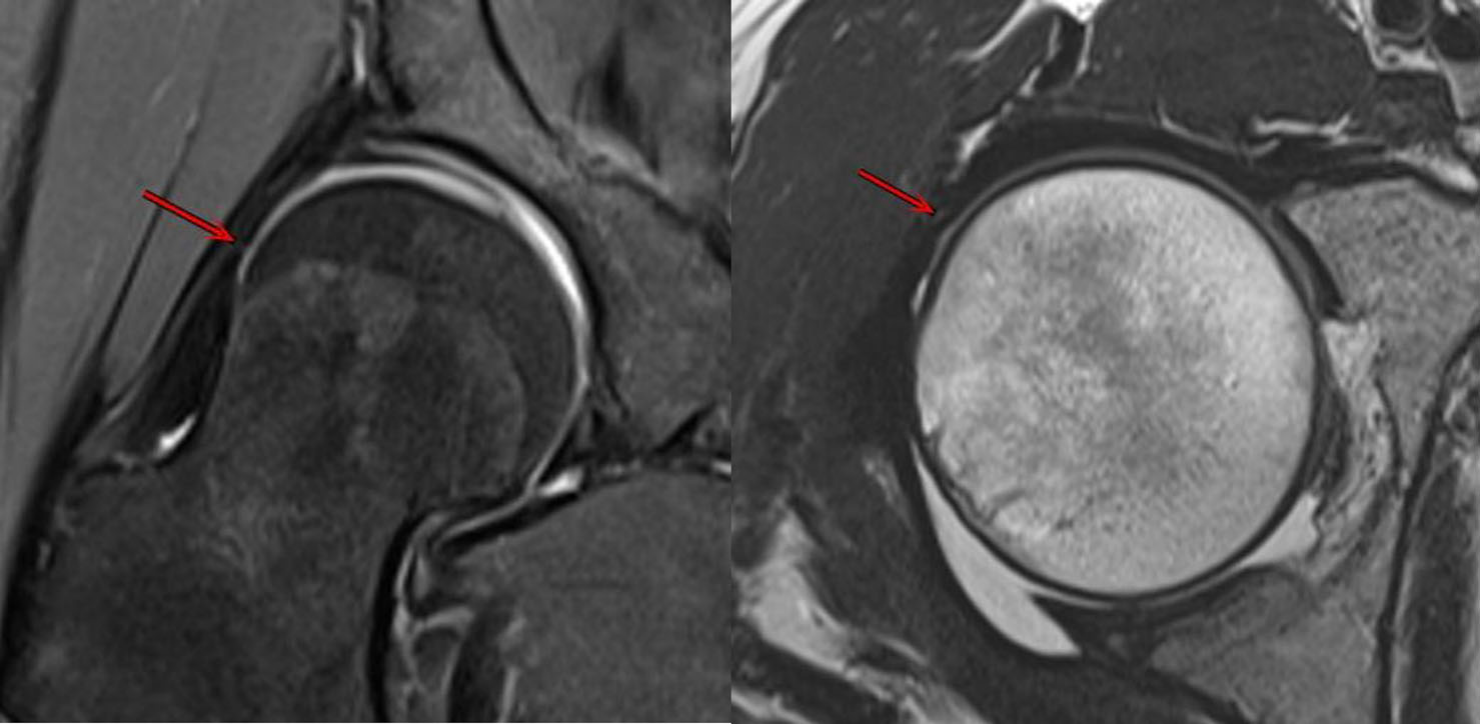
MRI shows 14 months after hip arthroscopy, the capsule has healed (red arrow).
